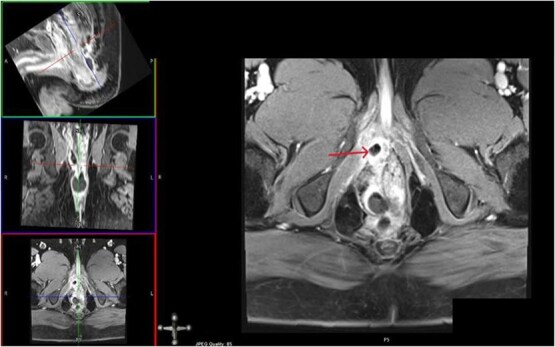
MRI of the anus revealing a small anterior abscess (arrow).
